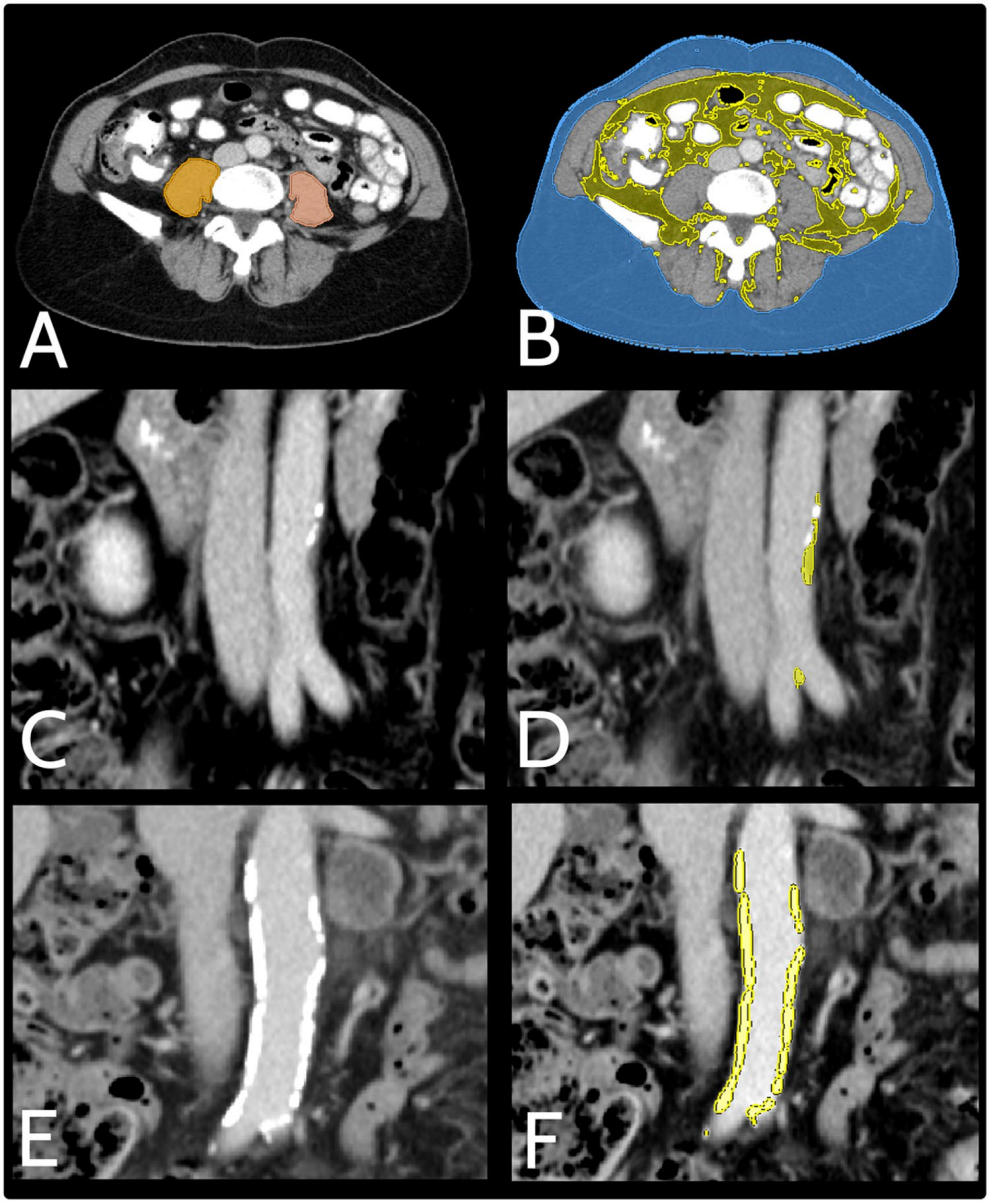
Example of applied image analysis. Portal-venous CT scans of the abdomen of a (A, B) 56-year-old female, (C, D) 64-year-old male and (E, F) 67-year-old male with malignant melanoma prior to immunotherapy with ipilimumab. (A) Semi-automatic segmentation of the psoas muscles in the axial plane at the L4 level for calculation of the mean psoas area and the mean psoas density. (B) Semi-automatic segmentation of the visceral and subcutaneous adipose tissue area in the axial plane at the L4 level with fat density thresholds set to −190 HU to −30 HU. (C–F) Three-dimensional segmentation of the soft (D) and hard (F) plaque volume compared to the non-segmented images (C, E). Mean applied density thresholds were 100 (range 60–100) HU and 185 (180–220) HU for soft and hard plaques, respectively.
